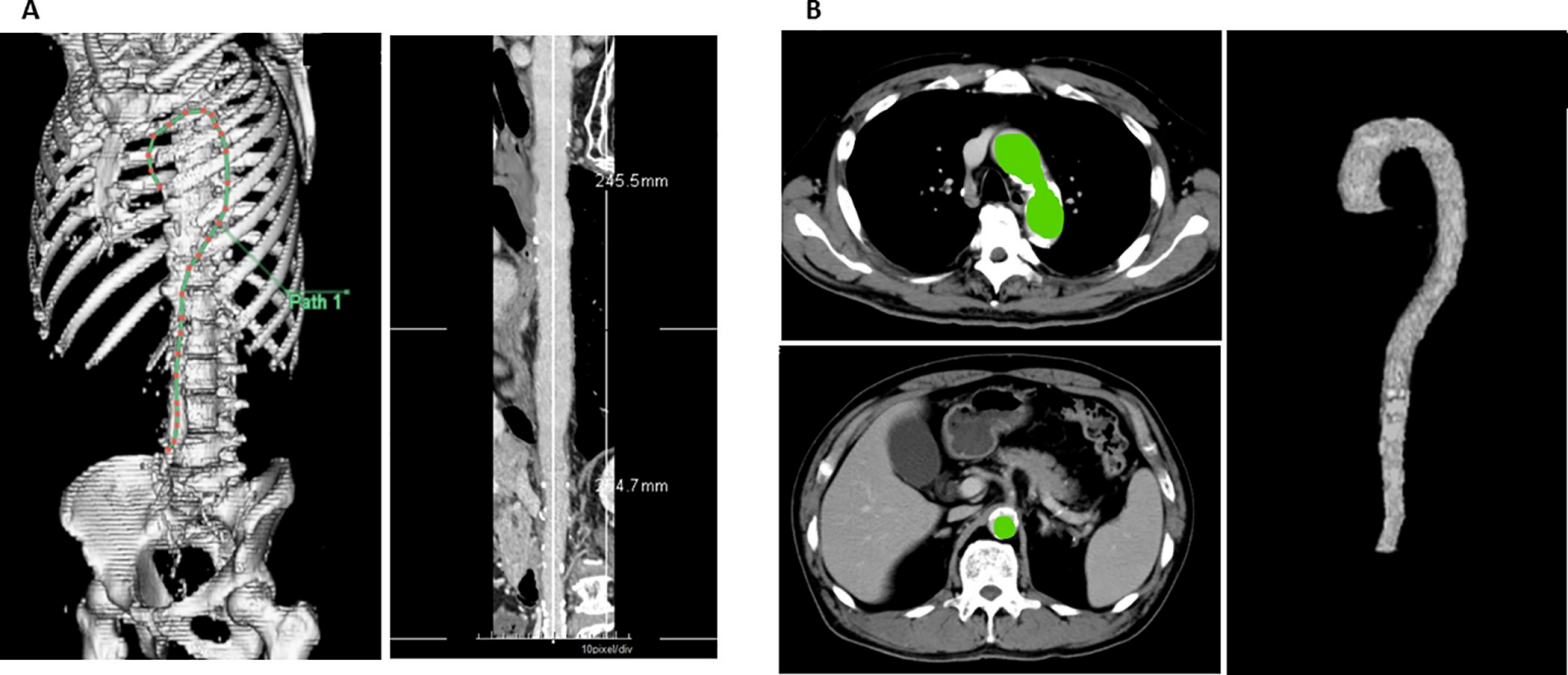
Measurement of the aortic length and volume with computed tomography. (A)The aortic length is measured by plotting the center of the vessel lumen from the ST junction to the aortic bifurcation. (B) The aortic volume is measured by tracing the arterial intima (in green) on about 20 slices from the ST junction to the aortic bifurcation on an axial-plane image, and then the volume is calculated using reconstructed data.
